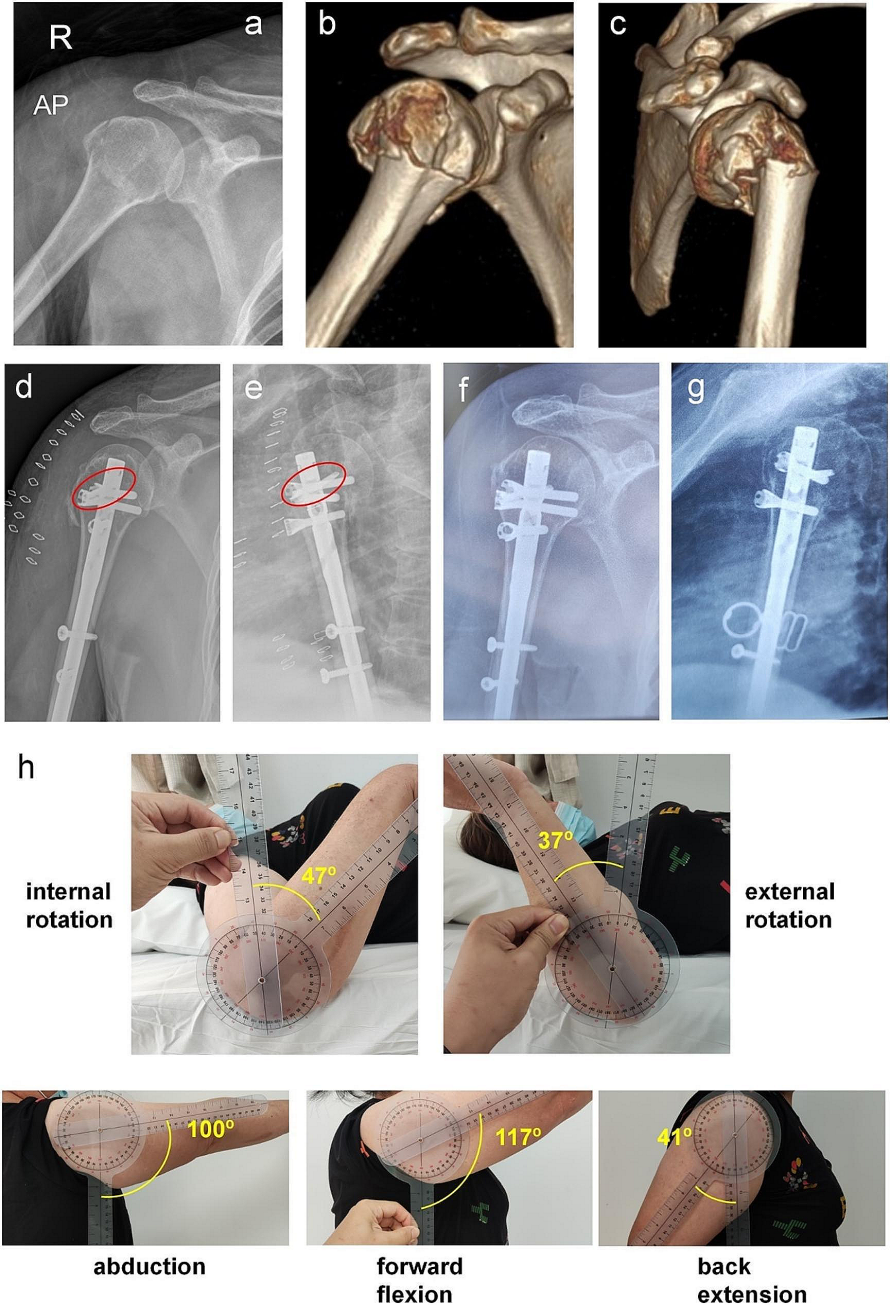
Female, 52 years old, three-part PHF. (a) Preop AP radiographs and (b, c) CT 3D reconstruction. Initial post-op (d and e) and 20 months post-op (f and g) showing fracture union without complication. (h) range of shoulder motion 20 months post-op
